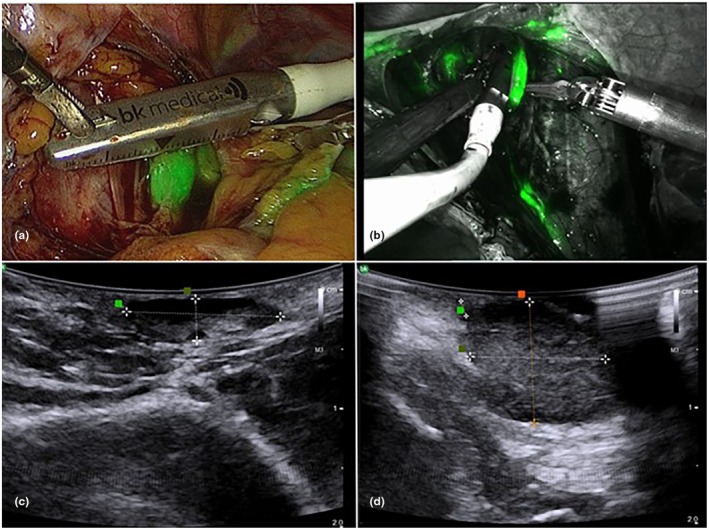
Intraoperative application of robotic ultrasound and representative findings. (a) Laparoscopic application of the ultrasound probe in indocyanine green (ICG) mode. (b) Robot assisted application of the ultrasound probe in ICG mode. (c) Image of a benign lymph node with long and short axis measurements. (d) Image of a malignant lymph node with long and short axis measurements.
